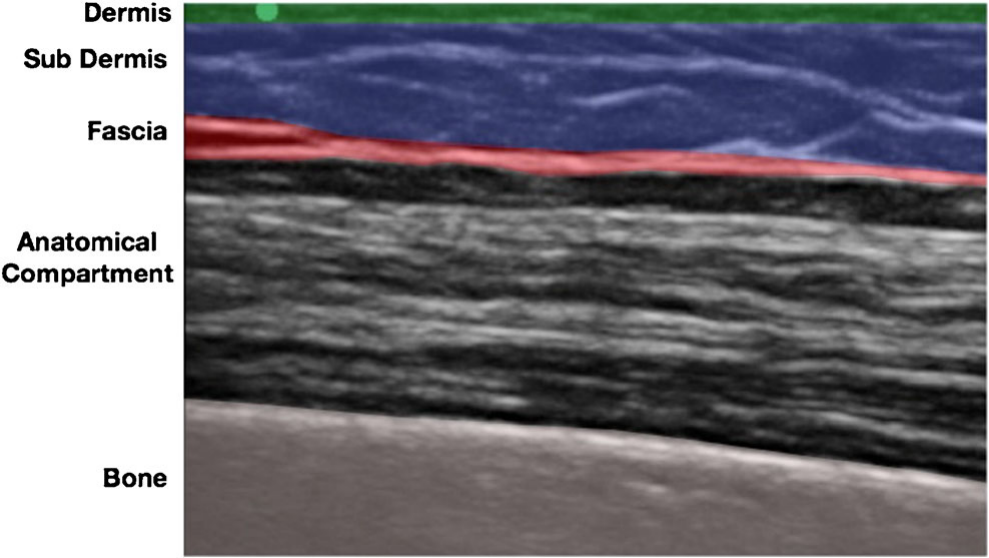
Longitudinal ultrasound image of the extensor compartment of the forearm of a soft embalmed human cadaver demonstrating the ultrasound appearances of key anatomical structures
